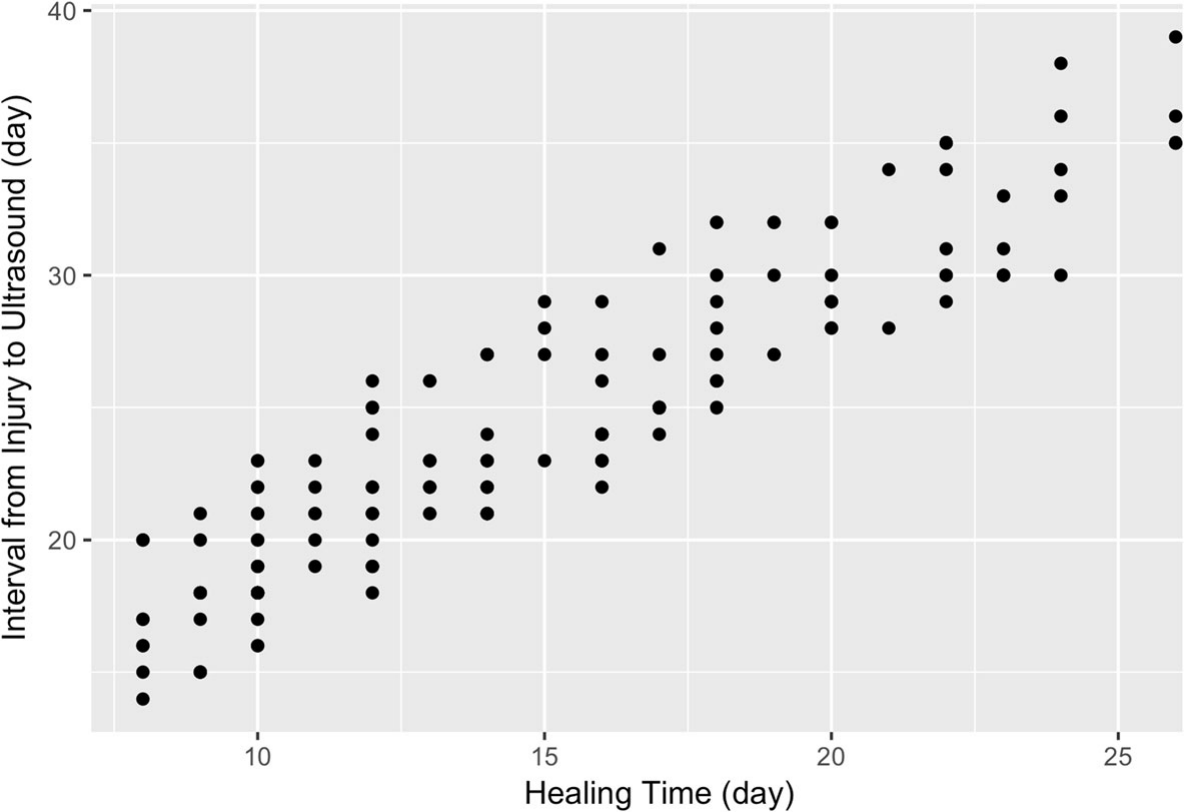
Time interval of the burn patients from injury date to ultrasound evaluation date. The mean was 24.28 days, and the standard deviation was 5.52 days
